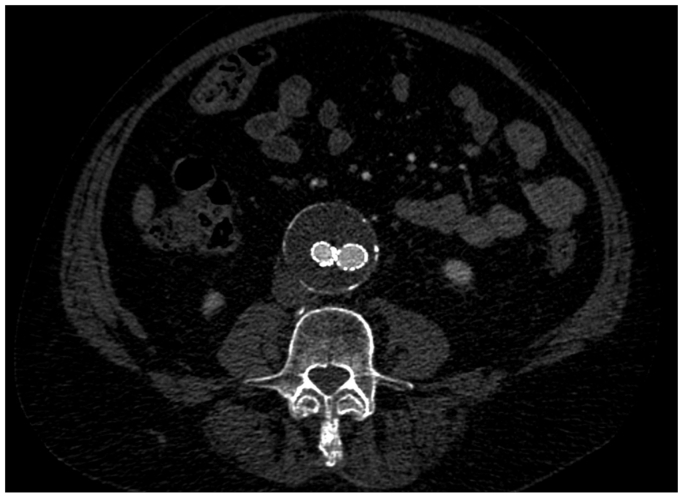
Computed tomographic angiography (CTA) reveals an excluded abdominal aortic aneurysm (AAA) without endoleaks.
